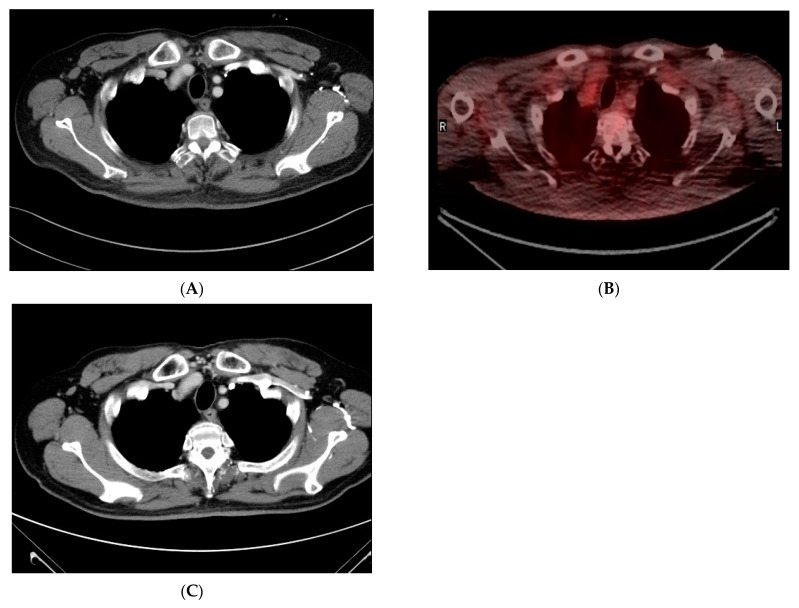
(A) After eight cycles of irinotecan and nivolumab, the follow-up CT scan showed a decrease in the size of the axillary lymph node and no residual mediastinal lymph nodes. (B) The follow-up PET-CT showed no F-18 fluorodeoxyglucose (FDG) avidity in right axilla, right lower para-tracheal region, bilateral hila, and left para-abdominal aortic region. (C) The patient had complete and durable response after four years of follow-up.
